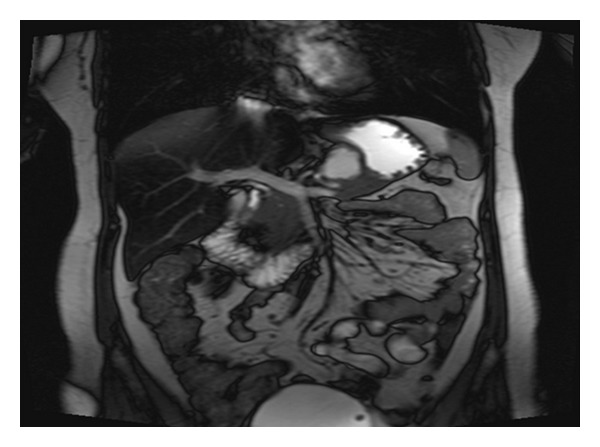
Abdominal MRI reveals a cystic lesion measured 4 × 2 cm in mid portion of pancreas.
